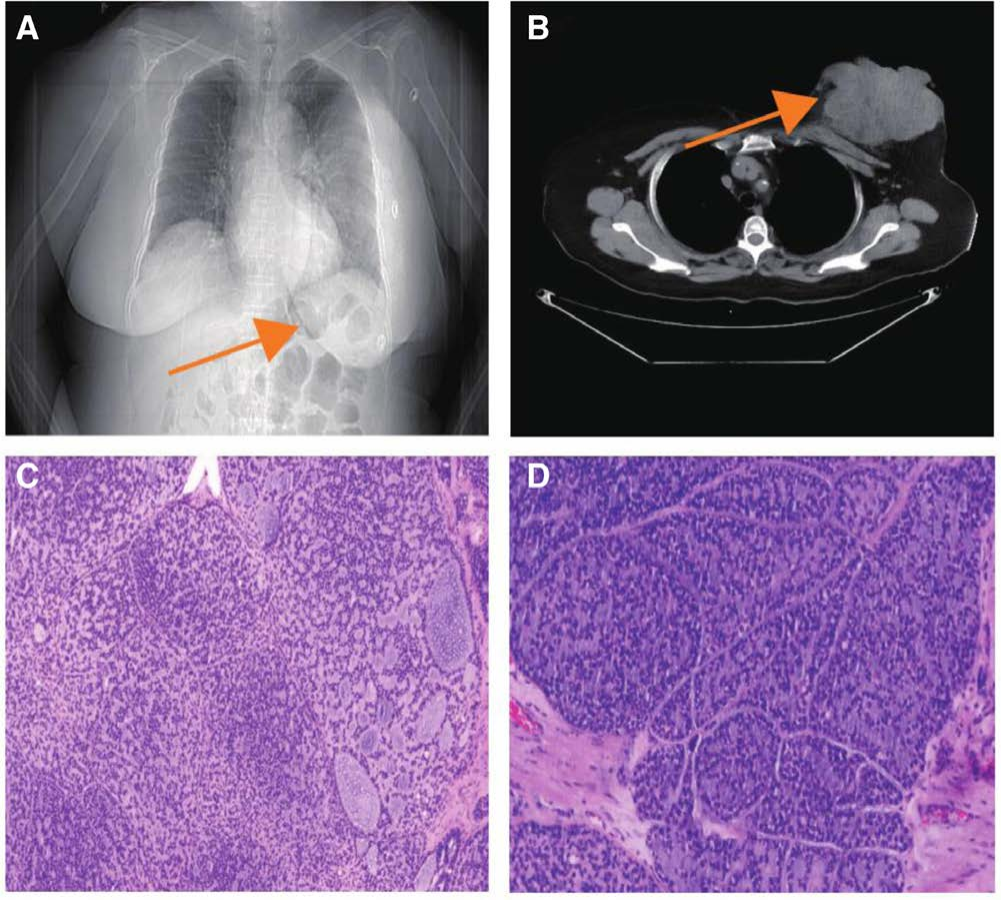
(A and B) Chest computed tomography findings: a large soft tissue mass of approximately 30 cm in diameter in the left breast. (C and D) Pathological findings of breast puncture.
